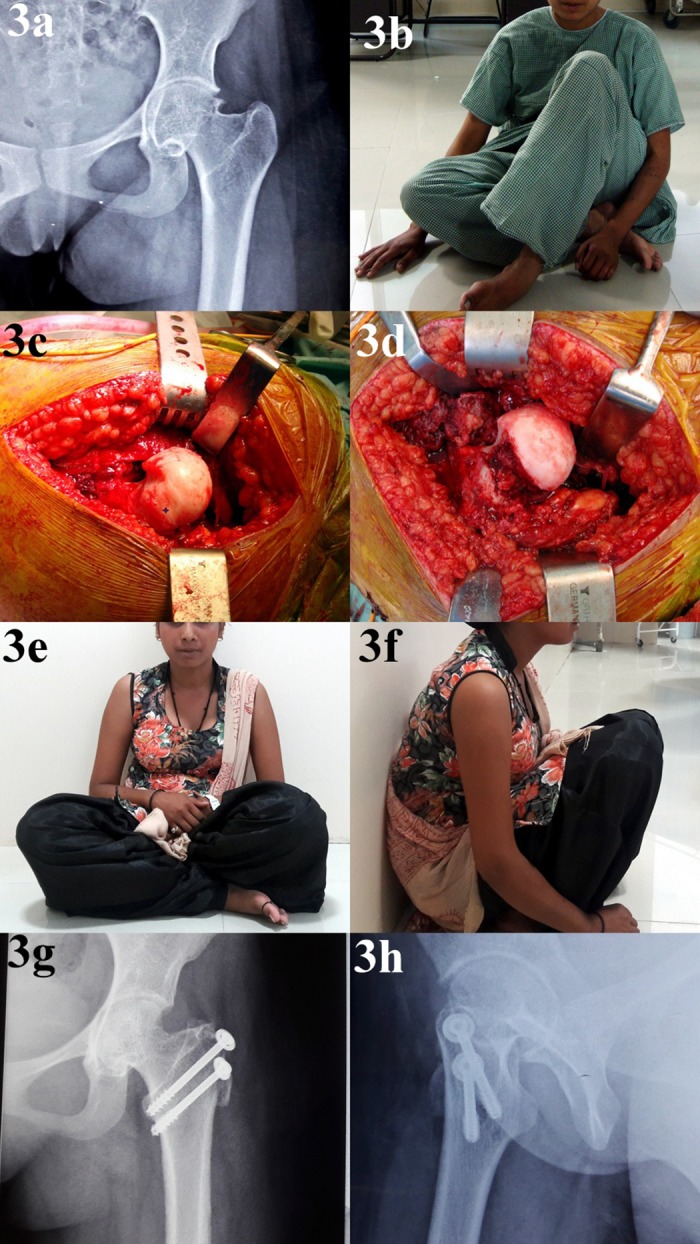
Details of a 27-year-old lady with idiopathic ONFH: panel a: preoperative radiograph showing cam deformity at femoral head–neck junction; panel b: she presented with painful restriction of flexion and internal rotation; panel c: intraoperative picture showing a large cam deformity. Femoral head cartilage looks normal; panel d: intraoperative image after femoral of cam deformity; panels e and f: follow-up pictures showing near normal hip range of motion. Floor-level activities such as squatting and sitting cross-legged are restored; panels g and h: post-operative radiograph showing normal appearing joint after removal of cam deformity.
